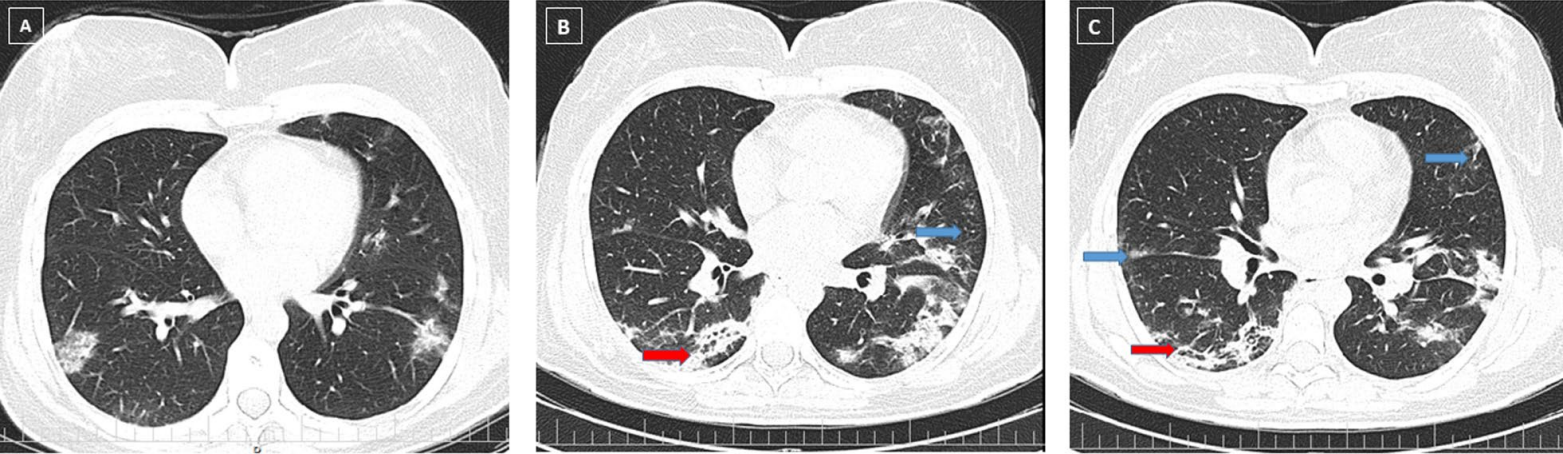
A 46-year-old female patient presented with fever and dyspnea, who was admitted to hospital and was discharged on day 15 of admission. Ground glass opacities and consolidations in several pulmonary lobes are observed in the initial CT scan (A). The total CT severity score was calculated as 14, and the disease severity was moderate. Three-month follow-up chest CT (B) shows some parenchymal bands and vacuolation (red arrow) and interlobular septal thickening (blue arrow) in several lobes. Six-month follow-up chest CT (C) shows a few parenchymal bands (red arrow) and interlobular septal thickening (blue arrow), which was diminished in comparison to the previous CT
